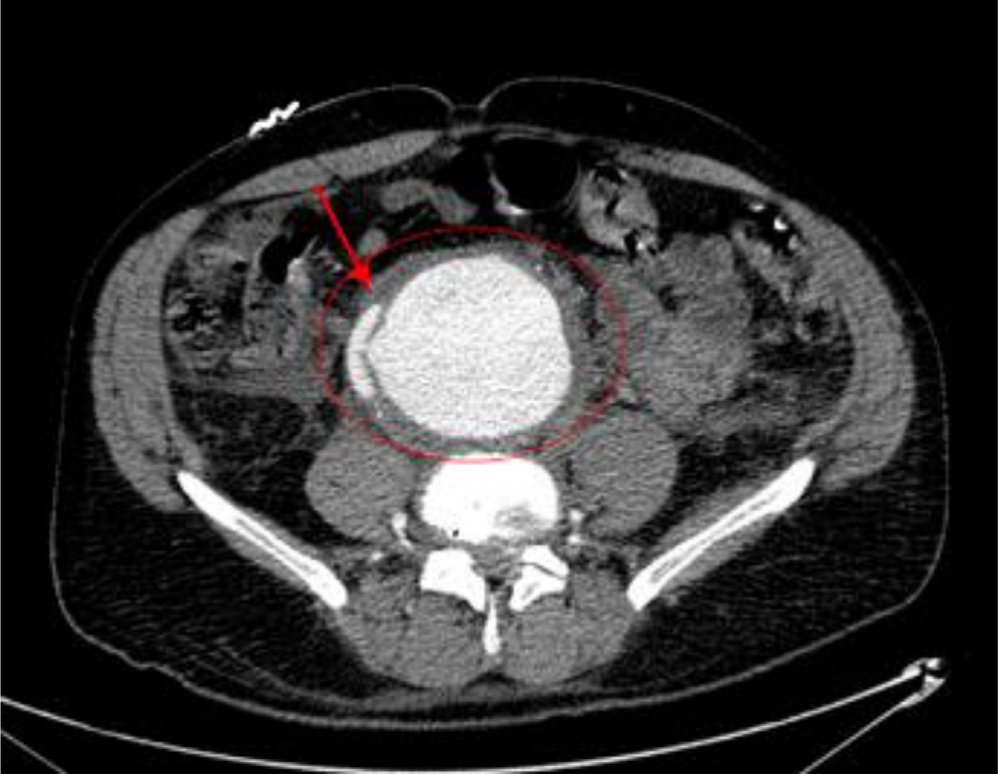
Axial CTA demonstrates fistulous tract between aorta and IVC.
